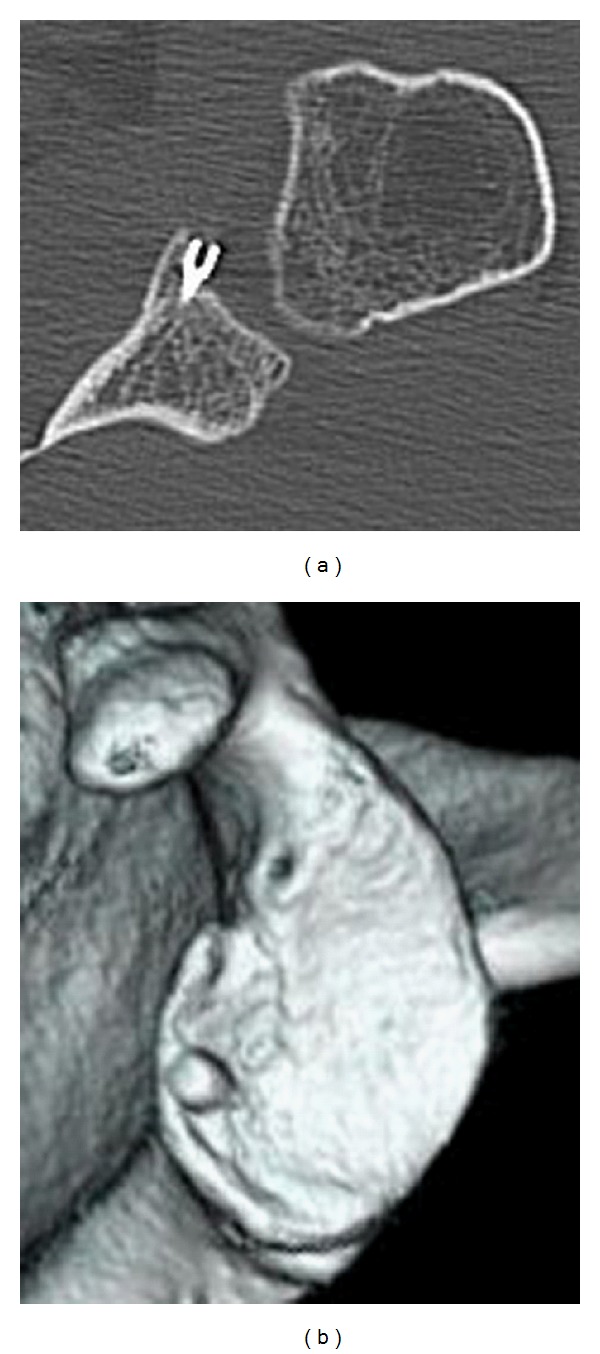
CT imaging at the time of revision. Axial image of CT showed union of the bony Bankart fragment, however, the screw end appeared to protrude intraarticular space (a). 3-dimensional CT (b).
